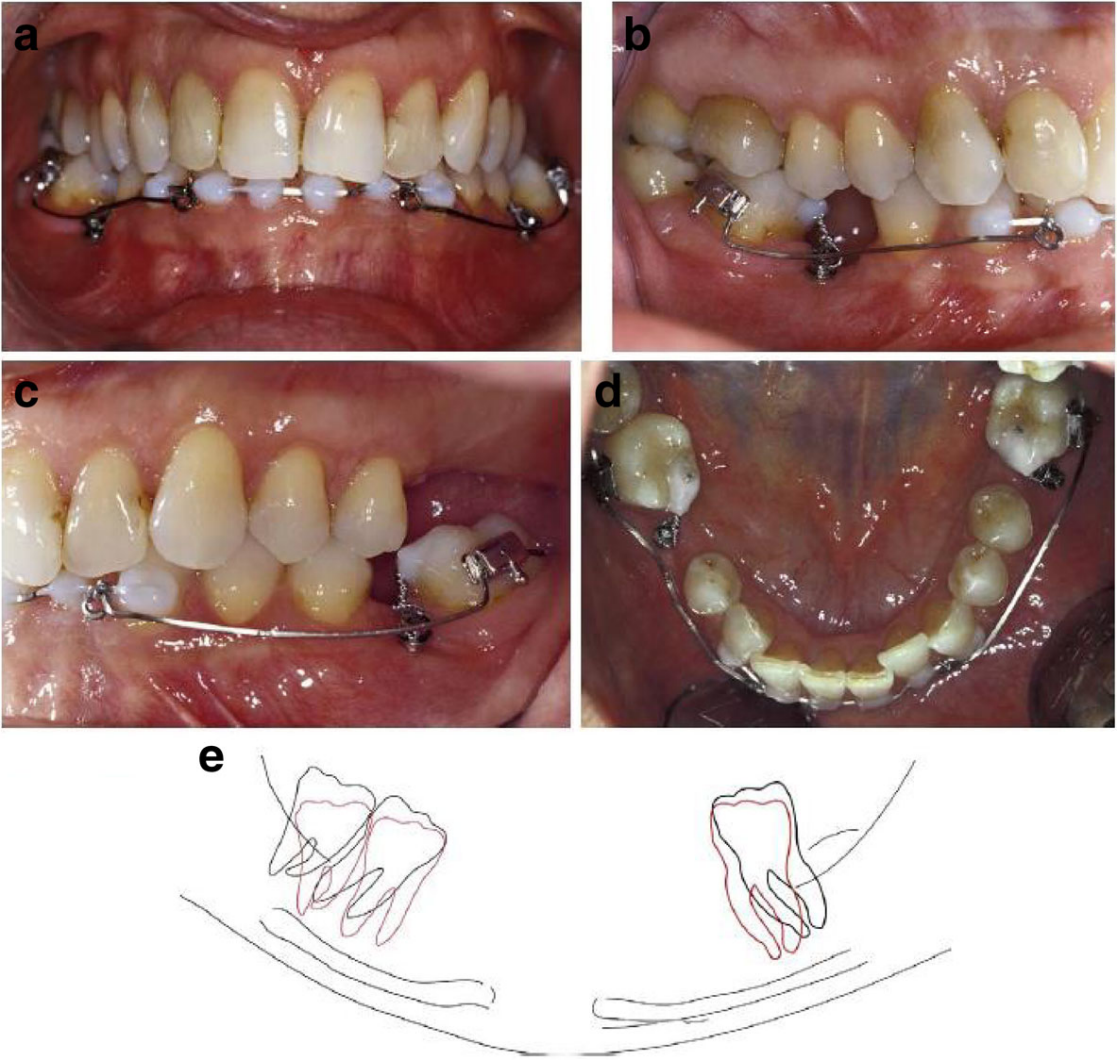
Uprighting of mesially tipped #37 and #46 with miniscrew implant and long cantilevers. The cantilevers generate molar uprighting, molar extrusion, and intrusion of the anterior teeth. Ligature between miniscrew and molar is used to control the extrusive force and the distal crown movement of each molar. Thus, the uprighting is mainly achieved by mesial root tipping. a The beginning of uprighting of #37 and #46 with long cantilevers, from the molar to the anterior teeth, and a screw mesial to the molar. b Lateral view at the initiation stage of uprighting of #46. c Lateral view at the initiation stage of uprighting of #37. d Occlusal view during the beginning stage of uprighting of #37 and #46. e Lateral view tracing of uprighting of #37 and #46 with superimposition. The black line stands for the initial position and the red line for the final position. The superimposition is applied on the panoramic radiograph and the mandibular canal the external oblique ridge and the lower border of the mandible are chosen as reference points (from Musilli et al. [2], with kind permission of Progress in Orthodontics)
